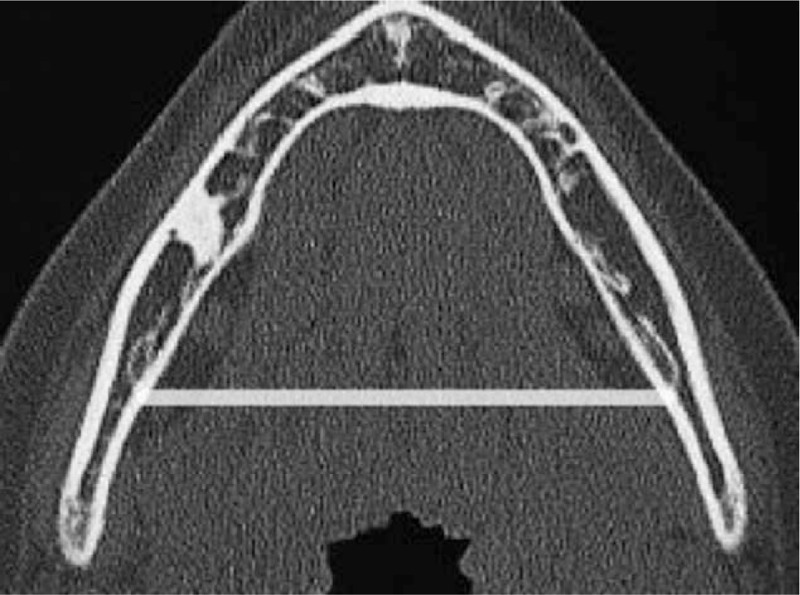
Axial measurement of inter-mandibular width via CT. CT = computed tomography.
